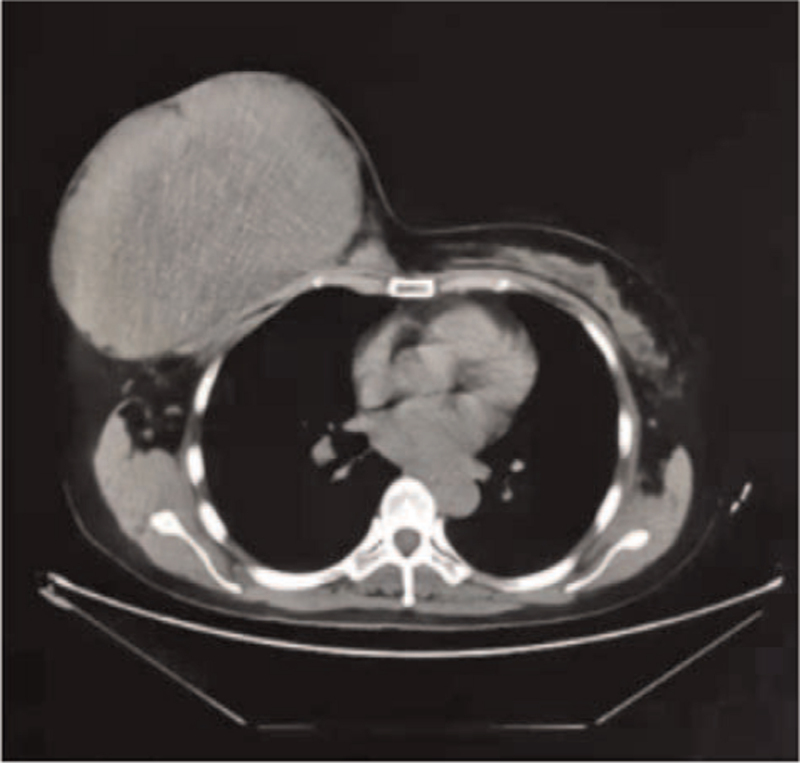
Chest CT showed a huge mass in the right breast.
